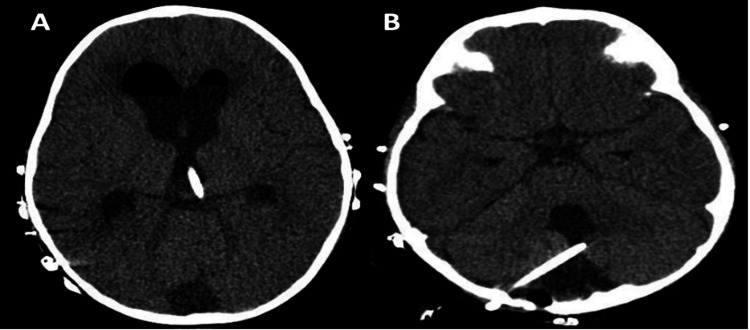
Postoperative CT brain scans. (A) Axial cut with marked reduced hydrocephalus changes and shunt in the lateral third ventricle; (B) axial cut with reduced posterior fossa size and the drain tip.
